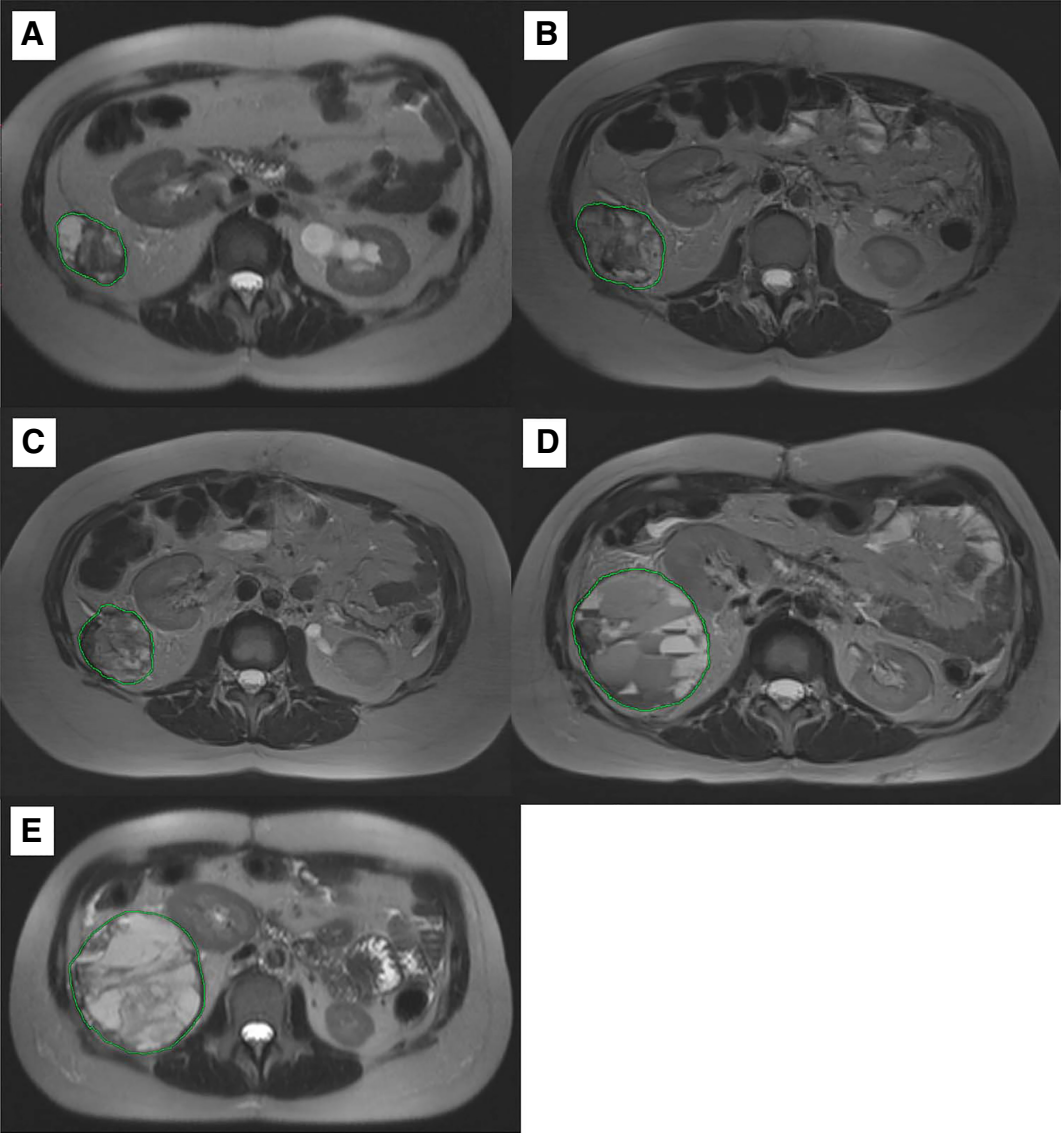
Cystic-solid neoplasm with tuberous contours in the right lateral channel (neoplasm #3). (*А*) MRI from 03.2016; (*B*) RI from 06.201; (*C*) MRI from 09.2016; (*D*) MRI from 10.2017; (*E*) MRI from 03.2018.
